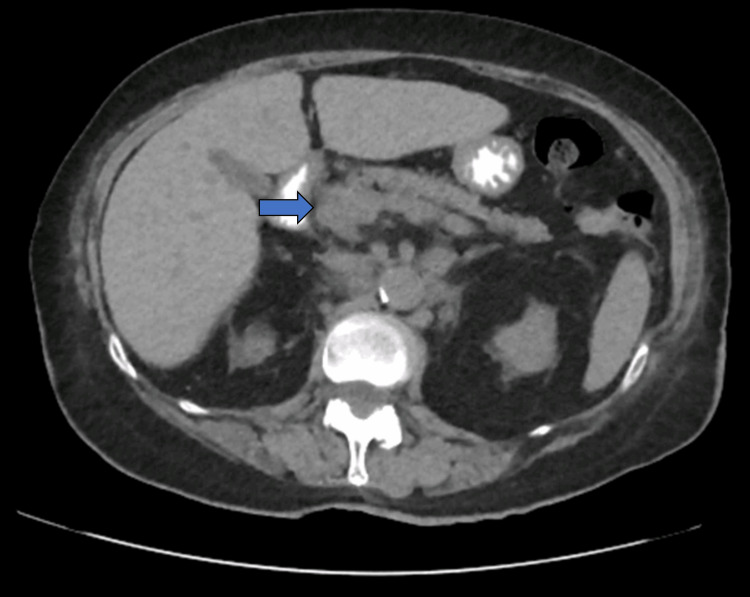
Abdominal CT scan showing the pancreas moderately atrophic with homogeneous parenchyma (blue arrow indicates the cephalic portion) Abbreviation: CT, computed tomography.
